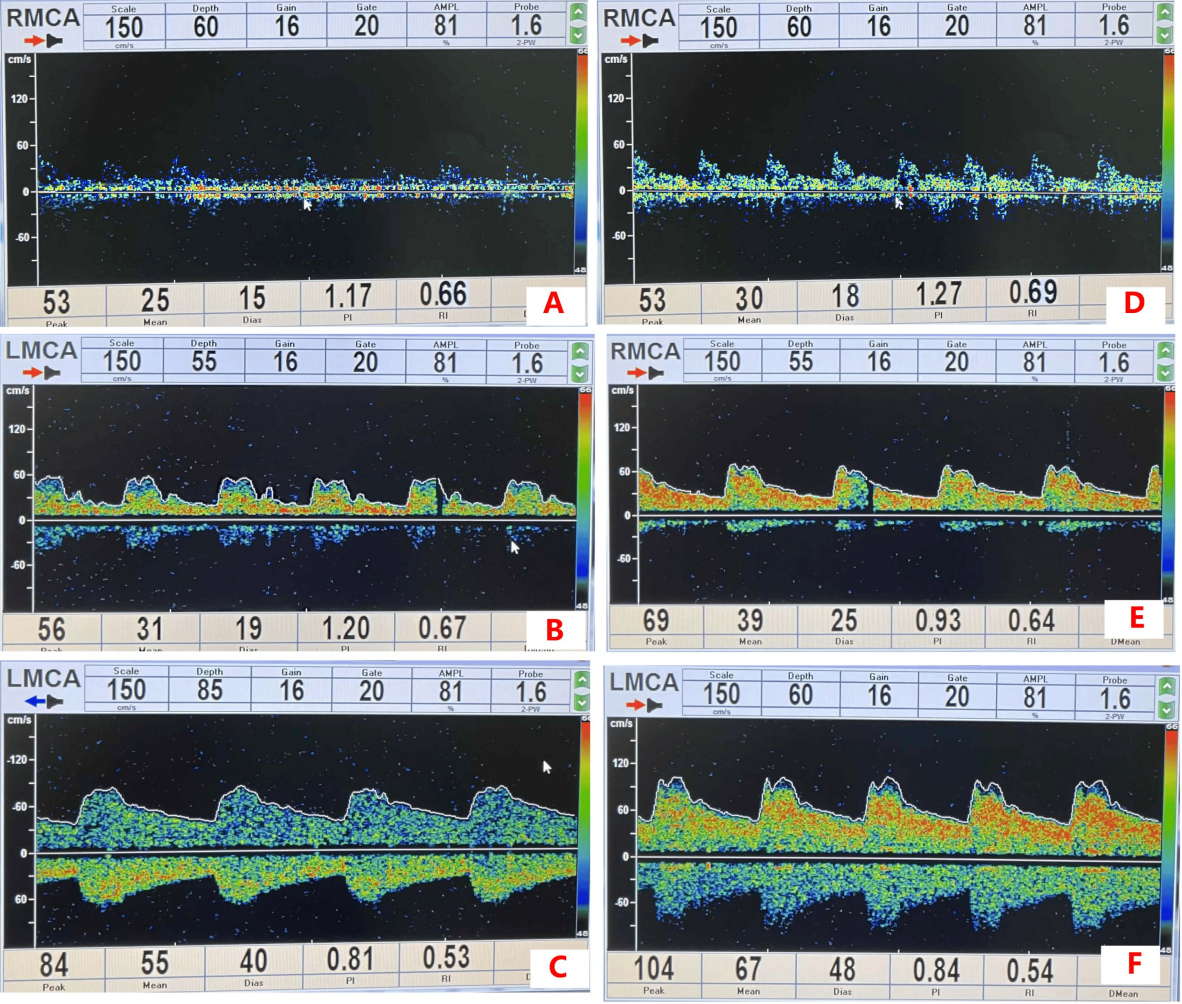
Samples of transcranial Doppler (TCD) flow images in the middle cerebral artery. (A-C) Flow images of group A at T3, T4, and T5. (D-F) Flow images of group B at T3, T4, and T5. LMCA: left middle cerebral artery; RMCA: right middle cerebral artery.
